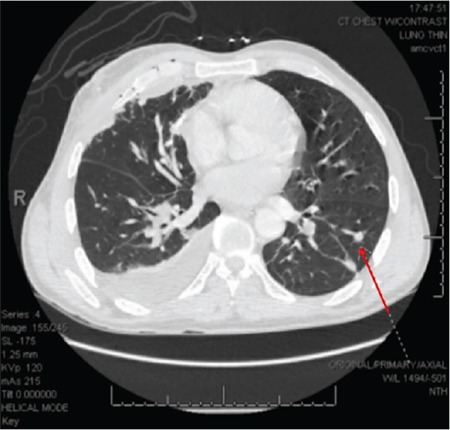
Axial computed tomography with intravenous contrast showing a pulmonary arteriovenous malformation in the left lower lobe measuring 7 mm.
